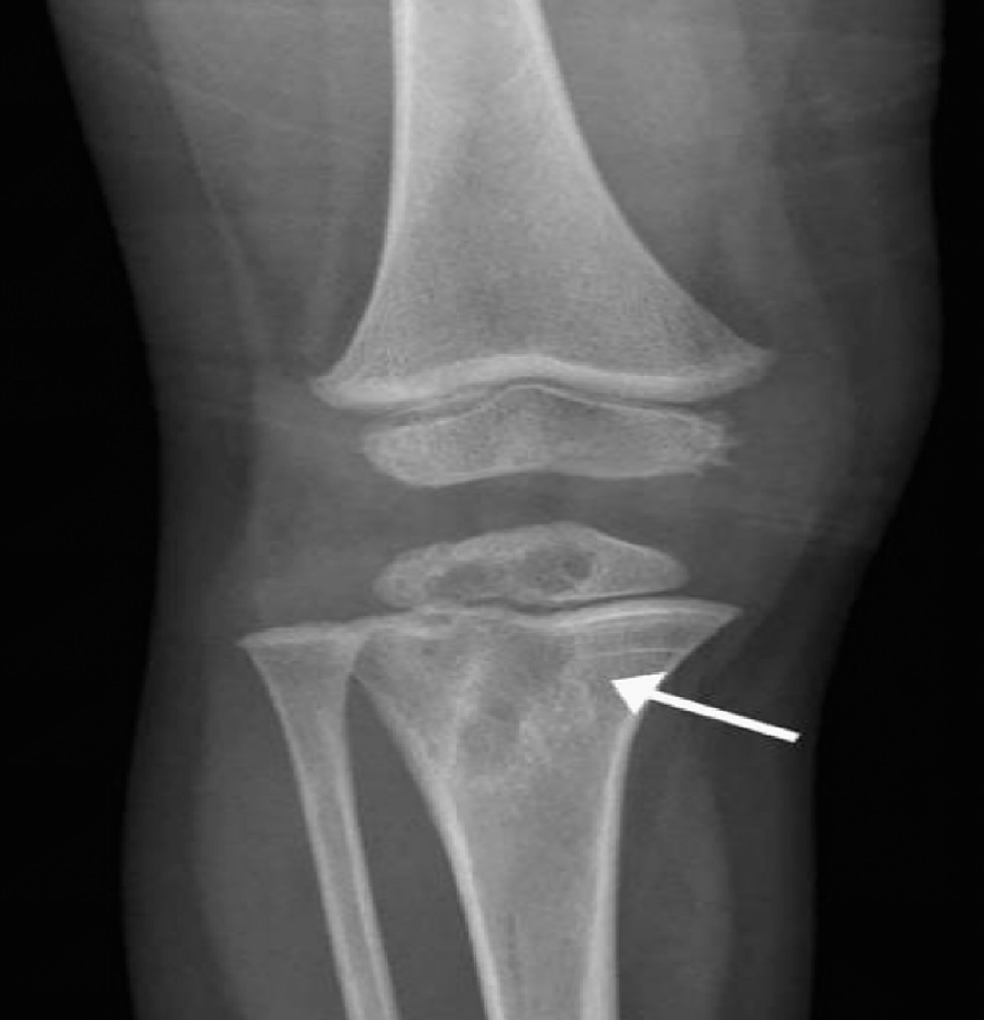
A 22-month-old girl with BCG osteomyelitis A plain radiograph shows an osteolytic lesion with well circumscribed sclerotic margin extending from the metaphysis, across the physis, and into the epiphysis [17].
